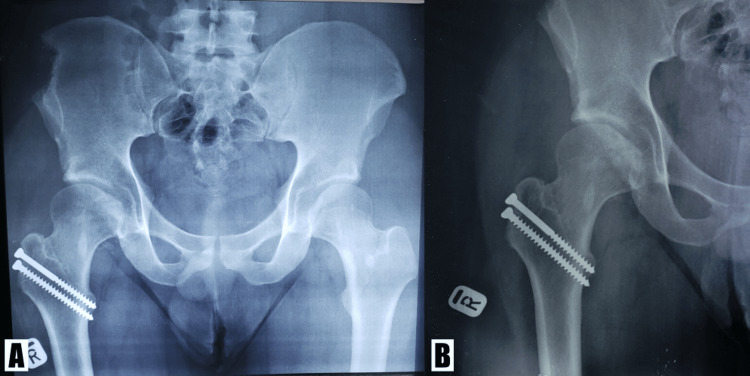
One-year post-operative plain radiographs (A) X-ray of the pelvis (anteroposterior view) and (B) right hip showing good bone union and shape of the femoral head, with no radiological evidence of AVN. AVN, avascular necrosis
